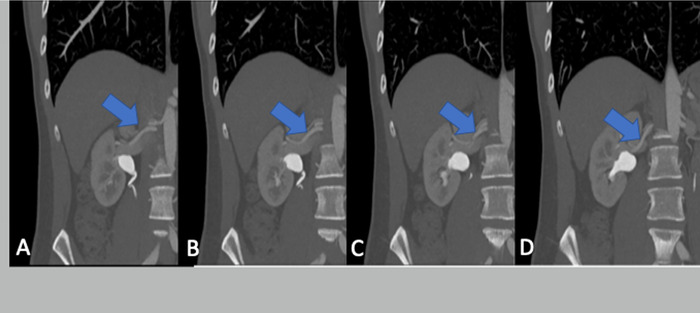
Coronal computed tomography angiography images of the donor's right kidney demonstrate complex extrarenal bifurcation (posterior to the inferior vena cava), crossing of 2 main arteries, and a short renal vein. Arrows point to the arteries. From left to right (A to D), the images become more posterior.
